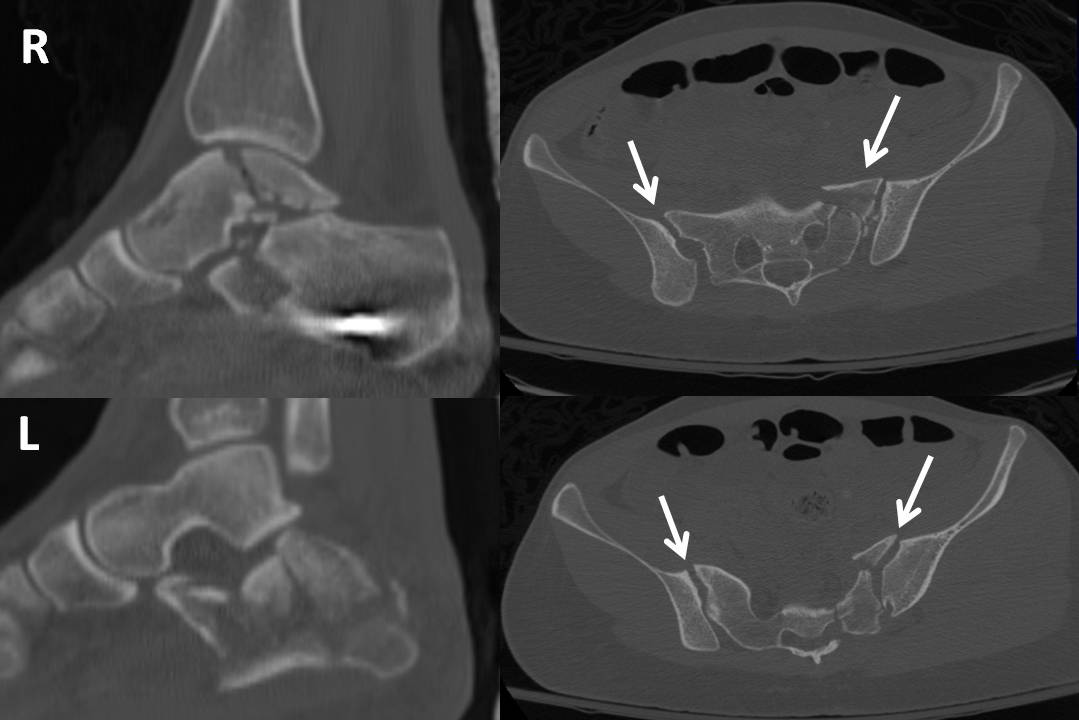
Injury pattern of bilateral lower extremities and pelvic fracture on initial multislice CT scan. The patient sustained a right-side open, comminuted talar body fracture, and a contralateral comminuted "joint-depression"-type calcaneus fracture, and a highly unstable pelvic ring injury with bilateral sacro-iliac joint disruptions (arrows).
